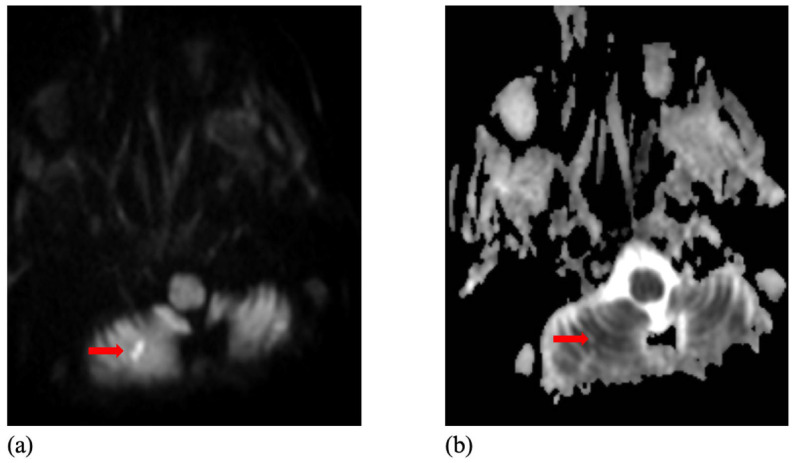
A Missed Ischemic Lesion by Artificial Intelligence on DWI and ADC Maps. A 92-year-old woman presented with acute-onset nausea, vomiting, and ataxia. The diffusion-weighted imaging (DWI) scan (a) and the apparent diffu-sion coefficient (ADC) map (b) showed an ischemic lesion in the right inferior cerebellum (arrows), which was not detected by the AI software.
